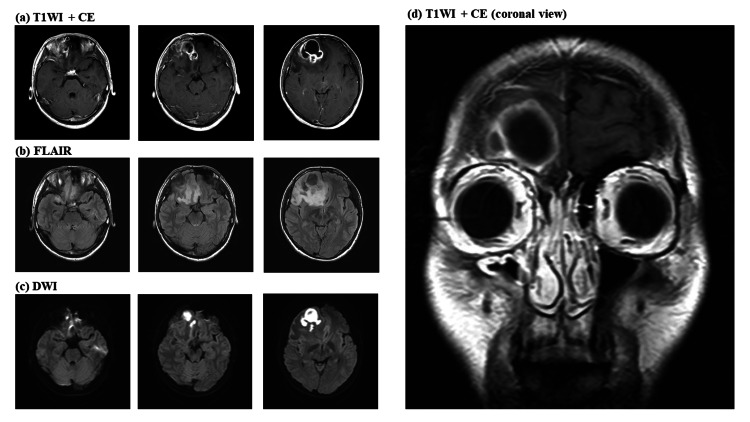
Initial MRI images of the patient who presented with a severe headache. (a) A ring-enhanced brain abscess measuring 40 mm was detected adjacent to the right frontal sinus on T1WI with contrast enhancement. (b) The brain abscess showed a mild right-to-left deviation with extensive FLAIR high signal around the lesion. (c) The brain abscess displayed a clear high signal on DWI. (d) Coronal T1WI with contrast enhancement revealed slight contrast findings at the site of the previous linear fracture. T1WI: T1-weighted image; CE: Contrast enhancement; FLAIR: Fluid-attenuated inversion recovery; DWI: Diffusion-weighted imaging.
